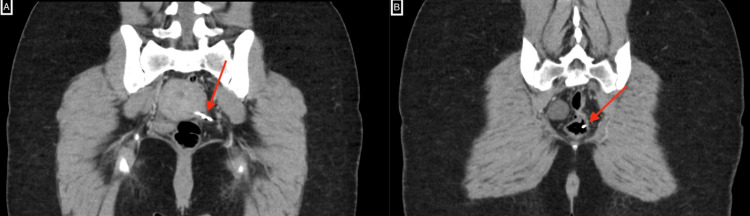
Contrast-enhanced CT of the pelvis showing the penetrating intrauterine device. The intrauterine device (red arrow) can be seen outside the endometrial cavity. Part of it can be seen penetrating the serosa of the uterus (A) and extending into the rectum (B).
